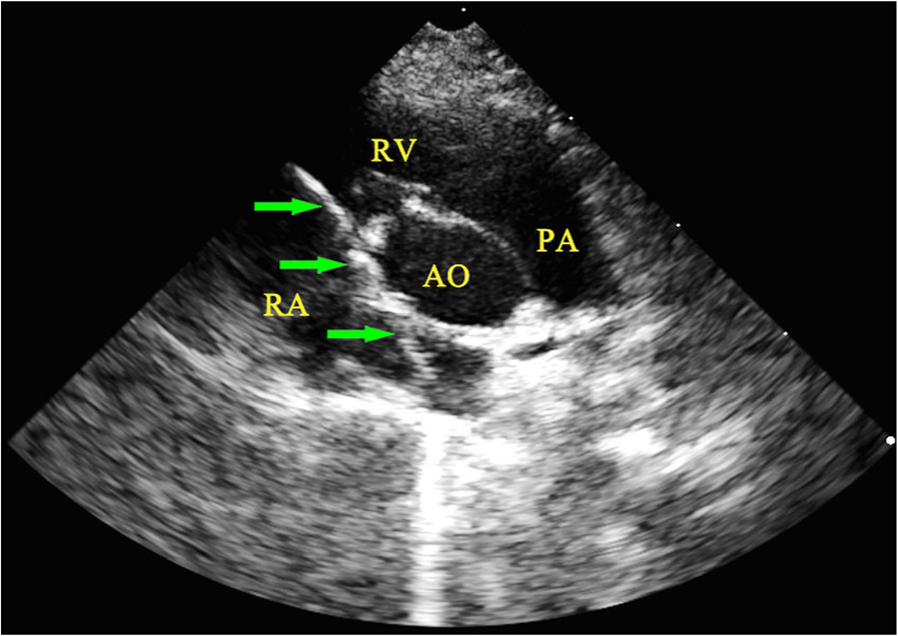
Passage of the catheter system through the tricuspid valve as visualized by echocardiography. The passage of the catheter system through the tricuspid valve and entering the right ventricle was visualized in aortic short axis view by echocardiography. The green arrows indicate the position of the catheter system. AO, aorta; PA, pulmonary artery; RA, right atrium; RV, right ventricle.
